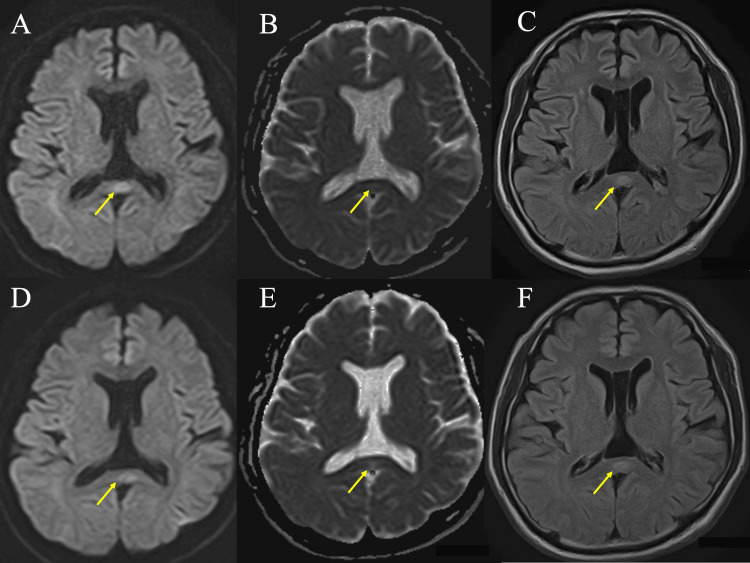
Case 3: MRI findings of CLOCCs (A) DWI shows a high-signal-intensity lesion in the corpus callosum (yellow arrow). (B) The ADC map shows no marked signal reduction in the corresponding region (yellow arrow). (C) FLAIR imaging shows high signal intensity in the affected region (yellow arrow). (D-F) Follow-up MRI shows marked improvement of the corpus callosum lesion, with no marked residual abnormality on DWI (D), slightly increased signal intensity on the ADC map (E), and faint residual hyperintensity on FLAIR imaging (F). ADC, apparent diffusion coefficient; CLOCCs, cytotoxic lesions of the corpus callosum; DWI, diffusion-weighted imaging; FLAIR, fluid-attenuated inversion recovery
